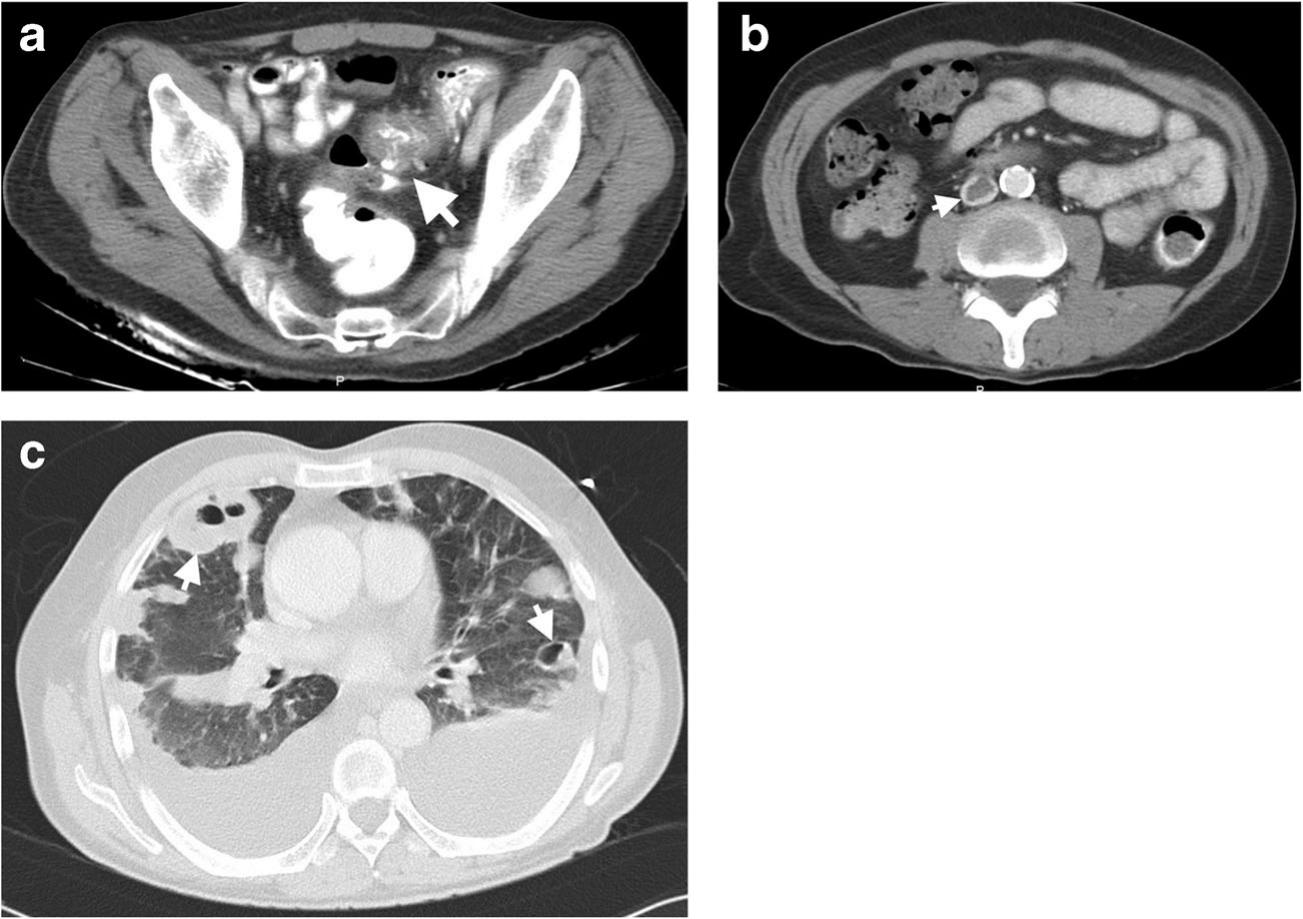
Pulmonary septic emboli in a 58-year-old male resulting from diverticulitis. a Axial contrast-enhanced CT of a 58 year-old man demonstrates edema and thickening of the sigmoid colon wall (*arrow*) with diverticulum and adjacent fat stranding. b Axial contrast-enhanced CT reveals thrombosis (*arrow*) in the inferior vena cava lumen. c Axial contrast-enhanced CT image at lung window setting shows multiple cavitary lesions (*arrows*) with thick wall representing septic emboli
